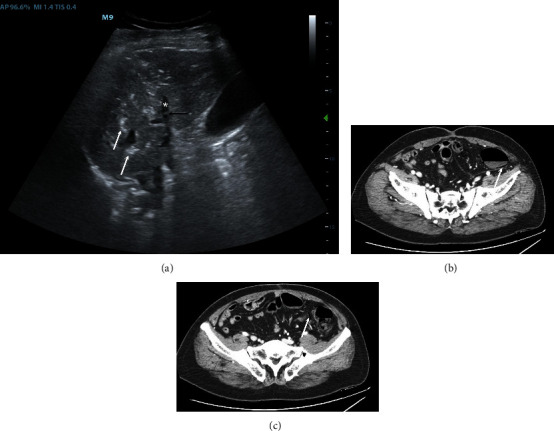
POCUS and CTA of case 2. (a) POCUS shows a right portal branch (asterisk) with some air bubbles into the lumen (black arrow). White arrows indicate some air in intrahepatic portal vessels. (b) CTA showing pneumatosis intestinalis (white arrow). (c) CTA shows the air flowing within the intestinal vessels (white arrow), as it is drained from the pneumatosis intestinalis, that will reach the portal vein. POCUS stands for point-of-care ultrasonography; CTA stands for computed tomographic angiography.
